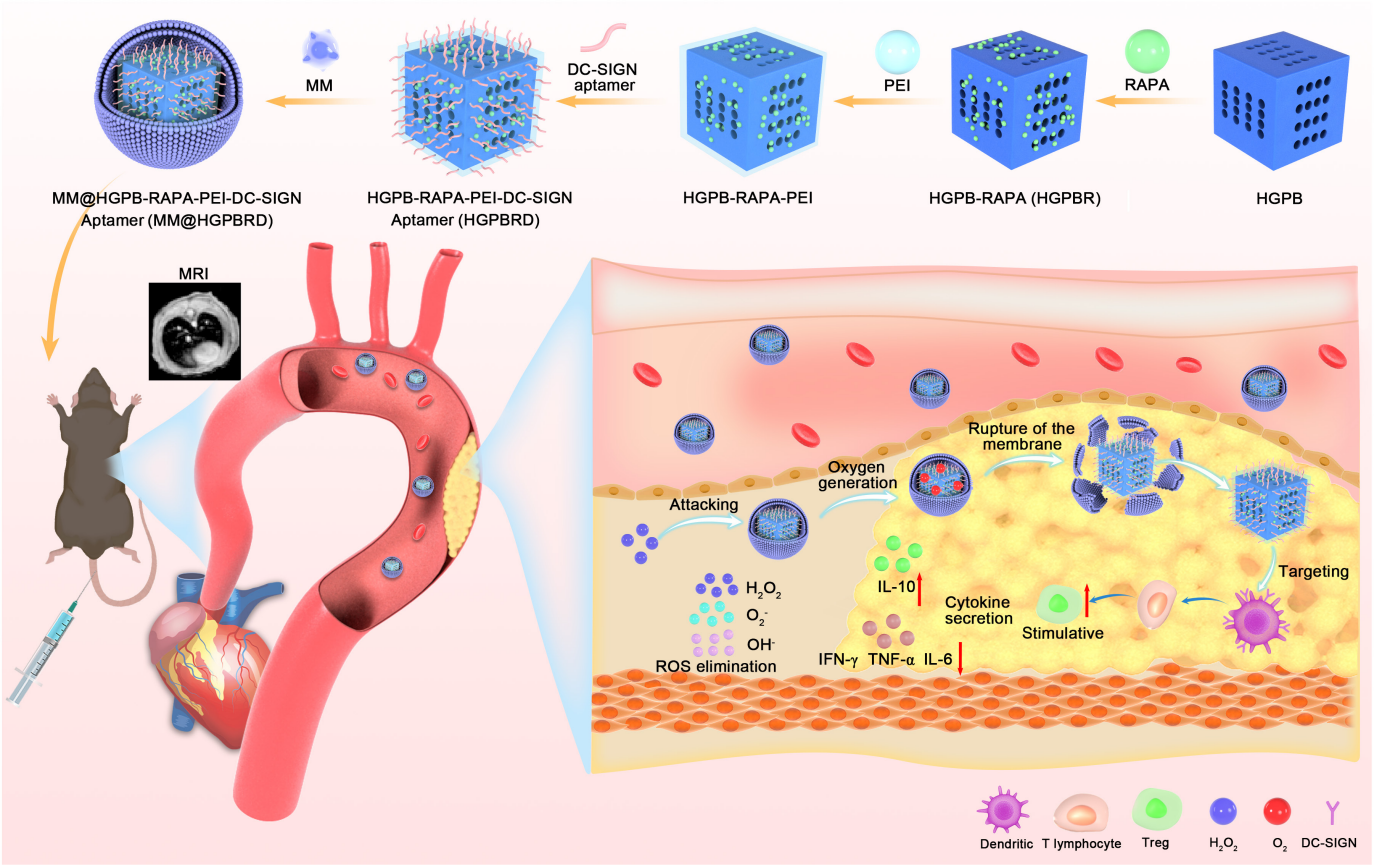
Preparation of MM@HGPBRD NPs for the inhibition and MRI of atherosclerosis.
